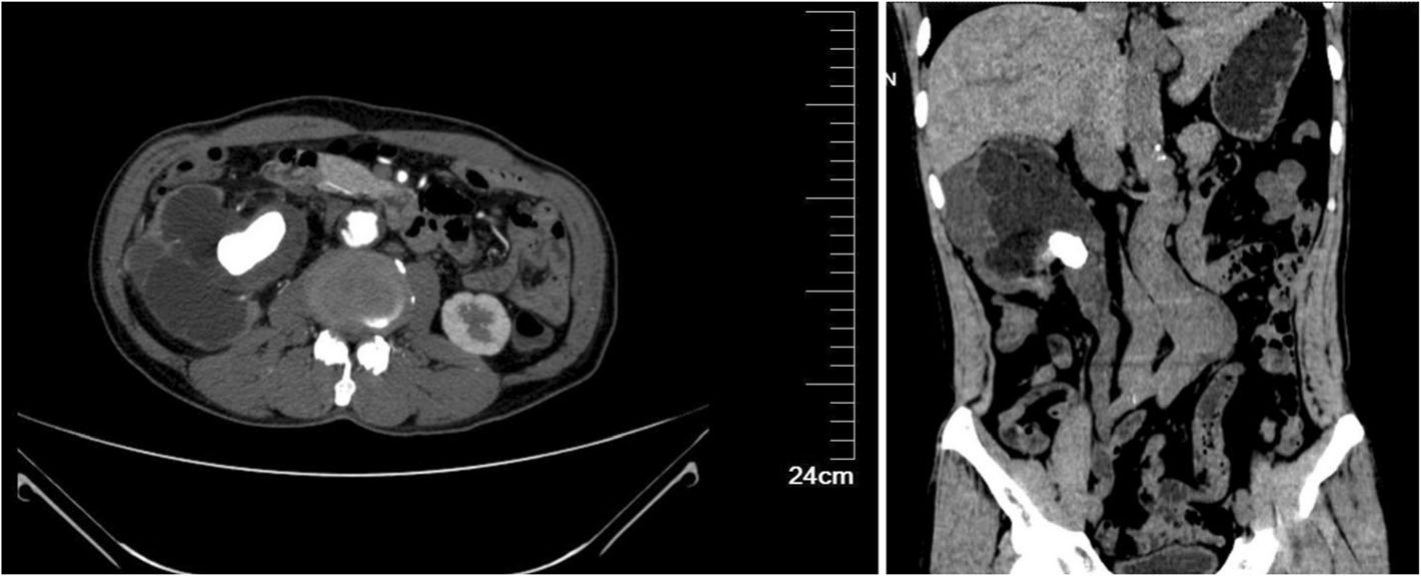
Abdominal CT demonstrated significant dilatation of the right renal pelvis and the right upper ureter with multiple calculi. The walls of the renal pelvis and the upper and mid-ureter were thickened with hyperdense soft tissue lesion
